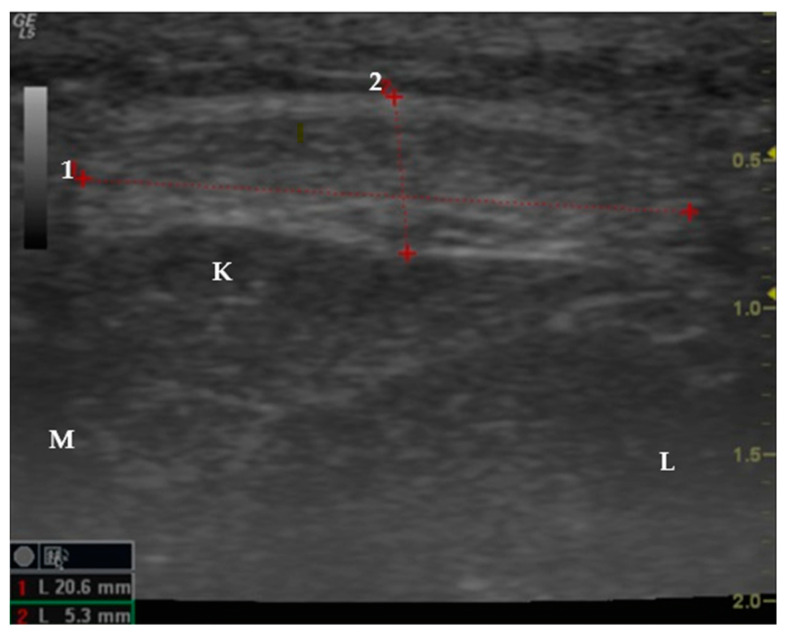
Transversal section of an ultrasound image showing the width (1) and thickness (2) measurements of the patellar tendon. K: Kager’s fat pad; L: lateral; M: medial.
